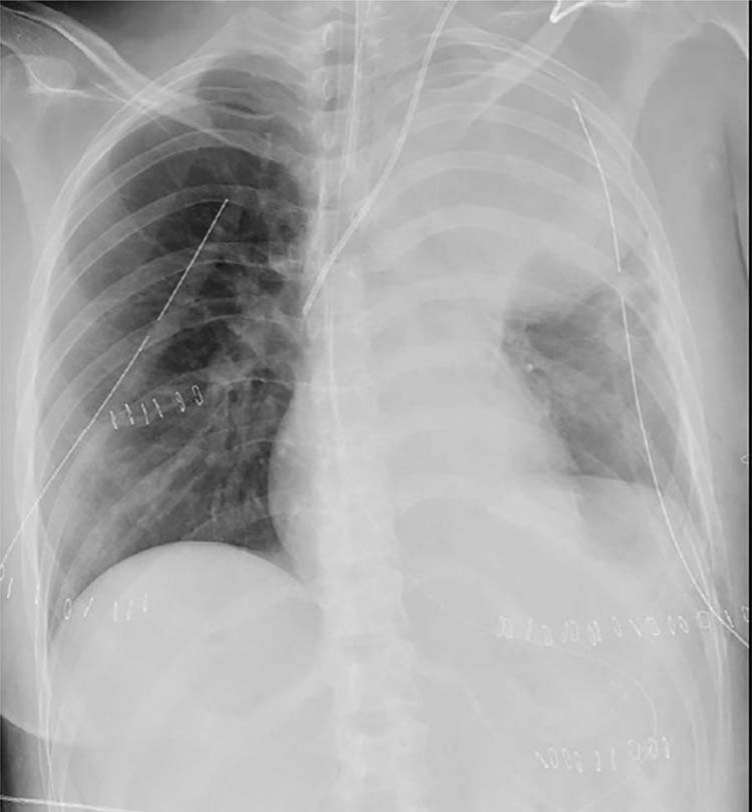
Chest roentgenogram of 2 days after initial emergency operation.
